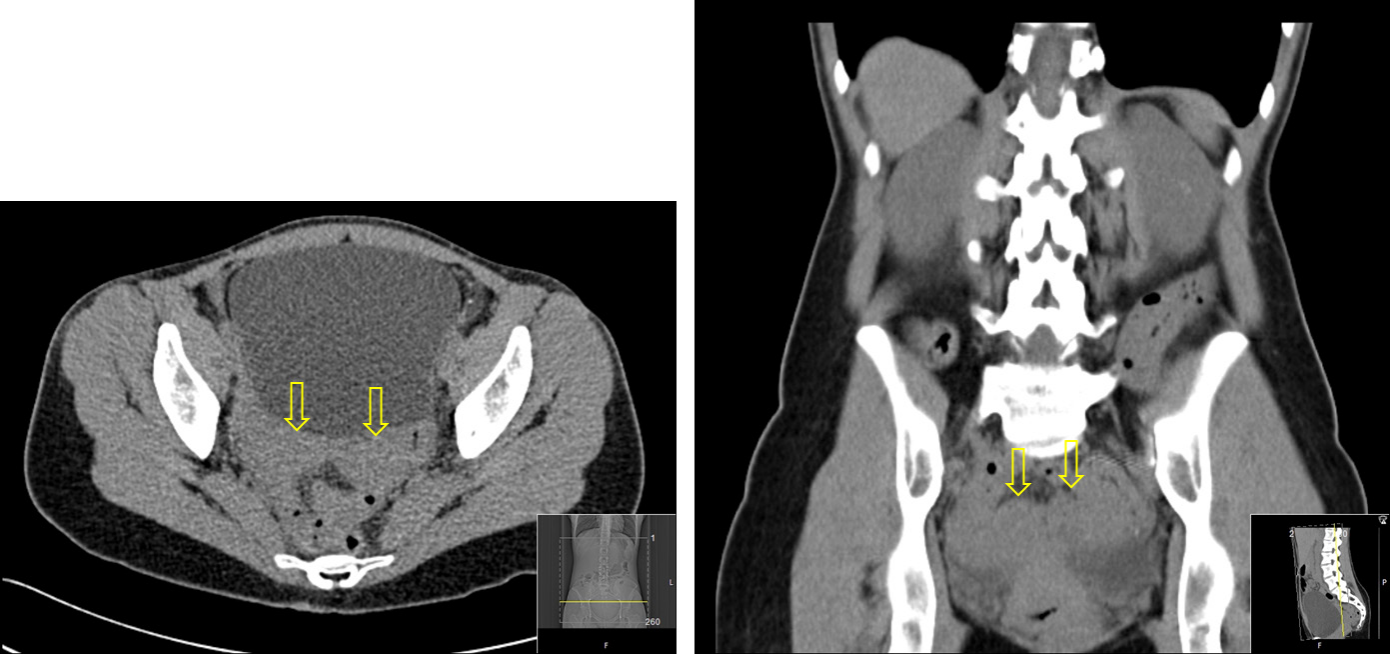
Abdominal CT demonstrating bicornuate uterus. Arrows indicate uterine ‘horns’.
